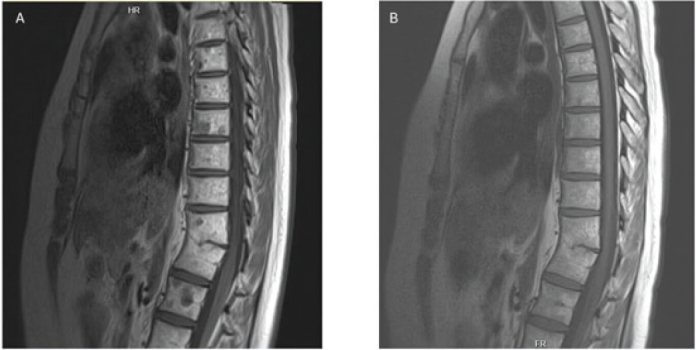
Spine magnetic resonance images. (A) In February 2015, MRI at detection of bone metastases with clear focal changes and (B) in October 2016, after 1.5 years of vismodegib therapy with no signs of lesion in corpus vertebrates. MRI: magnetic resonance imaging.
